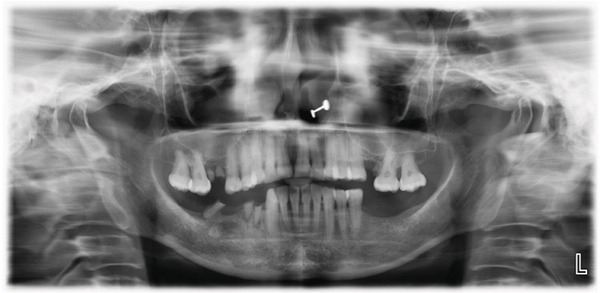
Radiographic presentation shown in Orthopantomogram.
